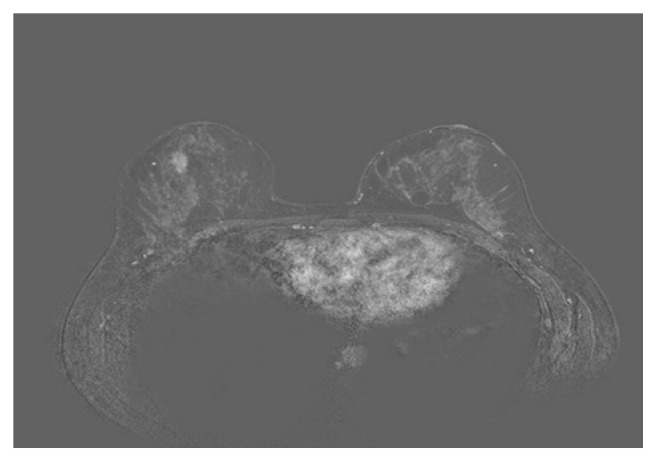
33-year-old high risk woman (prior history of irradiation to the chest). Screening MRI shows a focal nonmass enhancement in the right breast. Biopsy shows stromal fibrosis. Initial follow-up MRI after 6 months shows stable appearance. Further screening MRI scans show slight regression in size.
